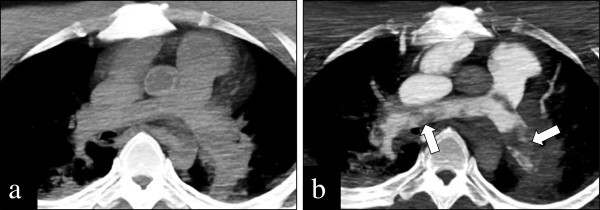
A 38-year-old man died for thromboembolism of pulmonary artery. Detection of thrombi is difficult with PMCT (a). On a resuscitation CEPMCT image, CT value of the pulmonary arterial trunk was 269 HU, and that of thromboemboli in the pulmonary artery was 54 HU. The filling defects in the pulmonary artery indicated the presence of thromboemboli (b).
